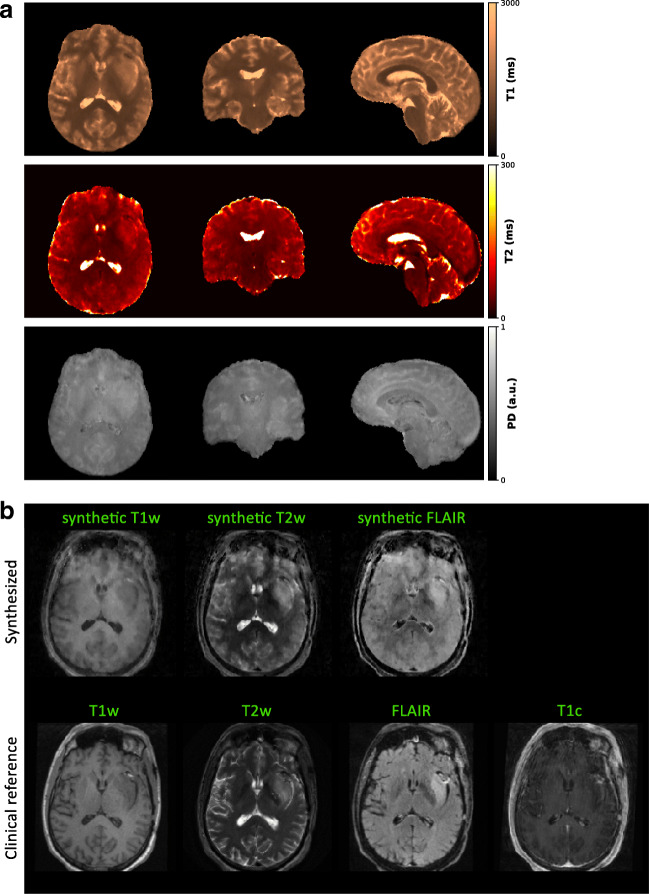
Sensitivity to rigid head motion. Profuse head motion can affect image acquisition in the transient-state, which leads to image degradation in the parametric maps (a) and the synthetic image contrasts (b) compared to the clinical contrast-weighted acquisitions. The post-contrast T1-weighted MRI indicates that state-of-the-art conventional MRI cannot fully recoup the pronounced head motion in this case. Corresponding sagittal and coronal views are shown in Fig. 12
